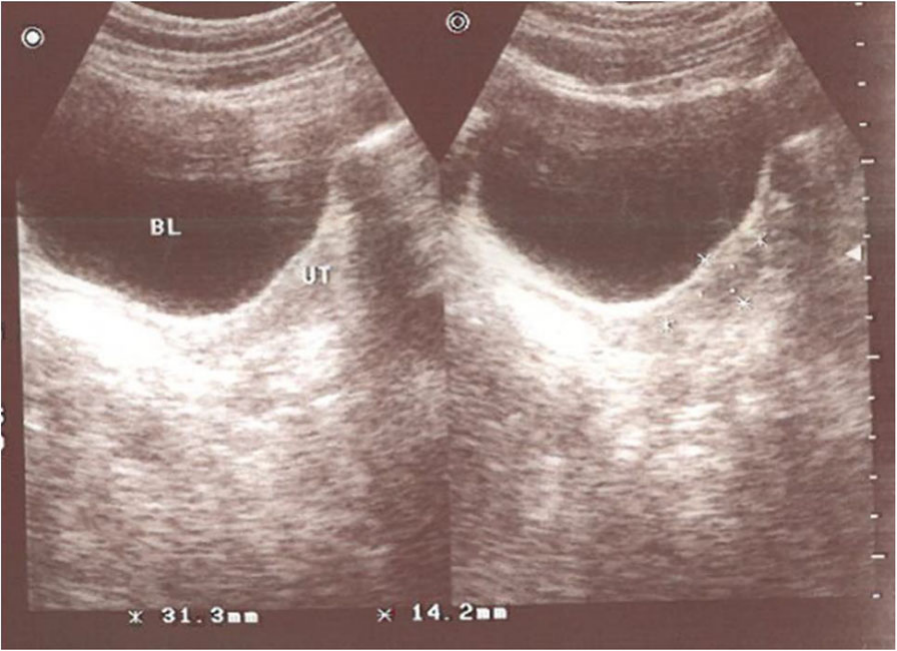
Ultrasound of patient’s uterus at 19 years of age. Uterus measures 31 × 14 mm
